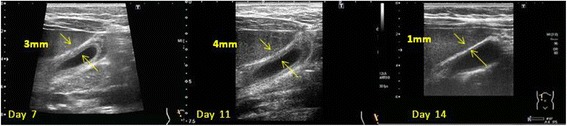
Ultrasonography of the gallbladder. Abdominal ultrasonography revealed a gallbladder without calculi, a thickened gallbladder wall (*arrows*), and perivesical fluid accumulation. These findings resolved by day 14 after admission
